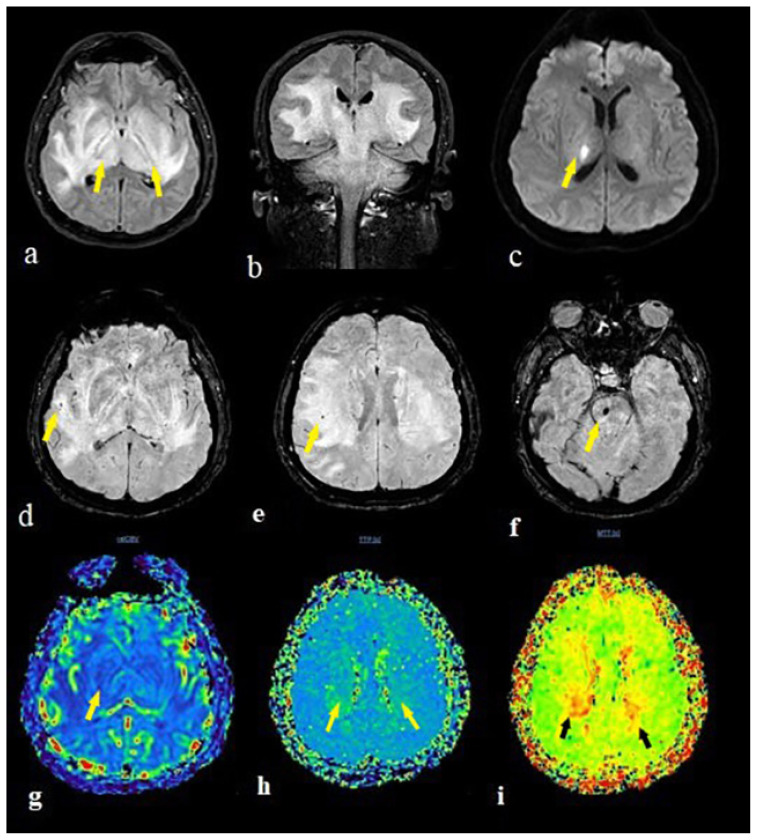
The brain MRI of a 41 years old male patient with encephalitis showed extensive hypertens signal in bilateral cerebral hemisphere white matter, thalamus, basal ganglia corticospinal tract, brain stem and serebellum on FLAIR images (a,b). The diffusion restrction occured only in the right thalamus on DWI sequence (c). There were microhemorrhages in the pons(f) and right parietal deep white matter (d,e) on SWI images. The perfusion MR imaging showed any CBV increment (g) with prolongation in bilateral thalamus and right temporal lobe on time to peak (h) and mean transit time (i) maps (arrows).
